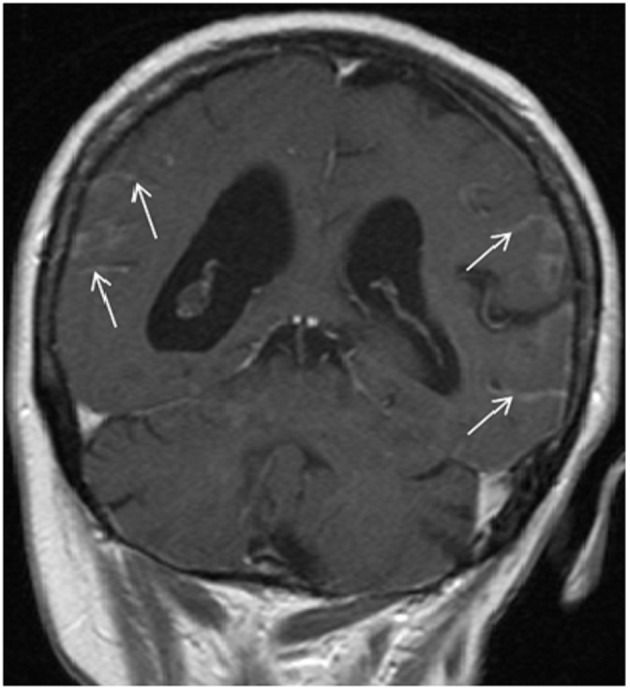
T1-weighted MRI image showing leptomeningeal enhancement (arrows).
